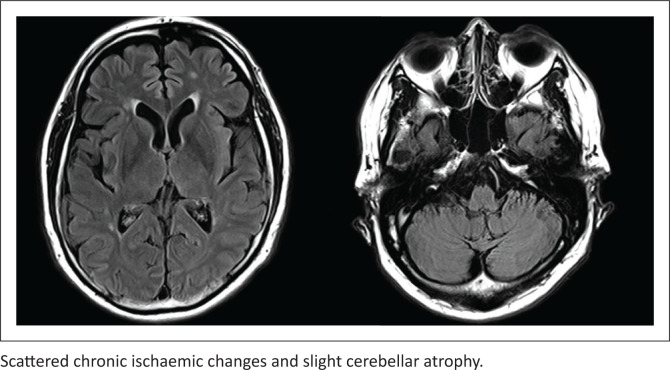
Axial Fluid-Attenuated Inversion Recovery (FLAIR) images of the brain on day 242.
